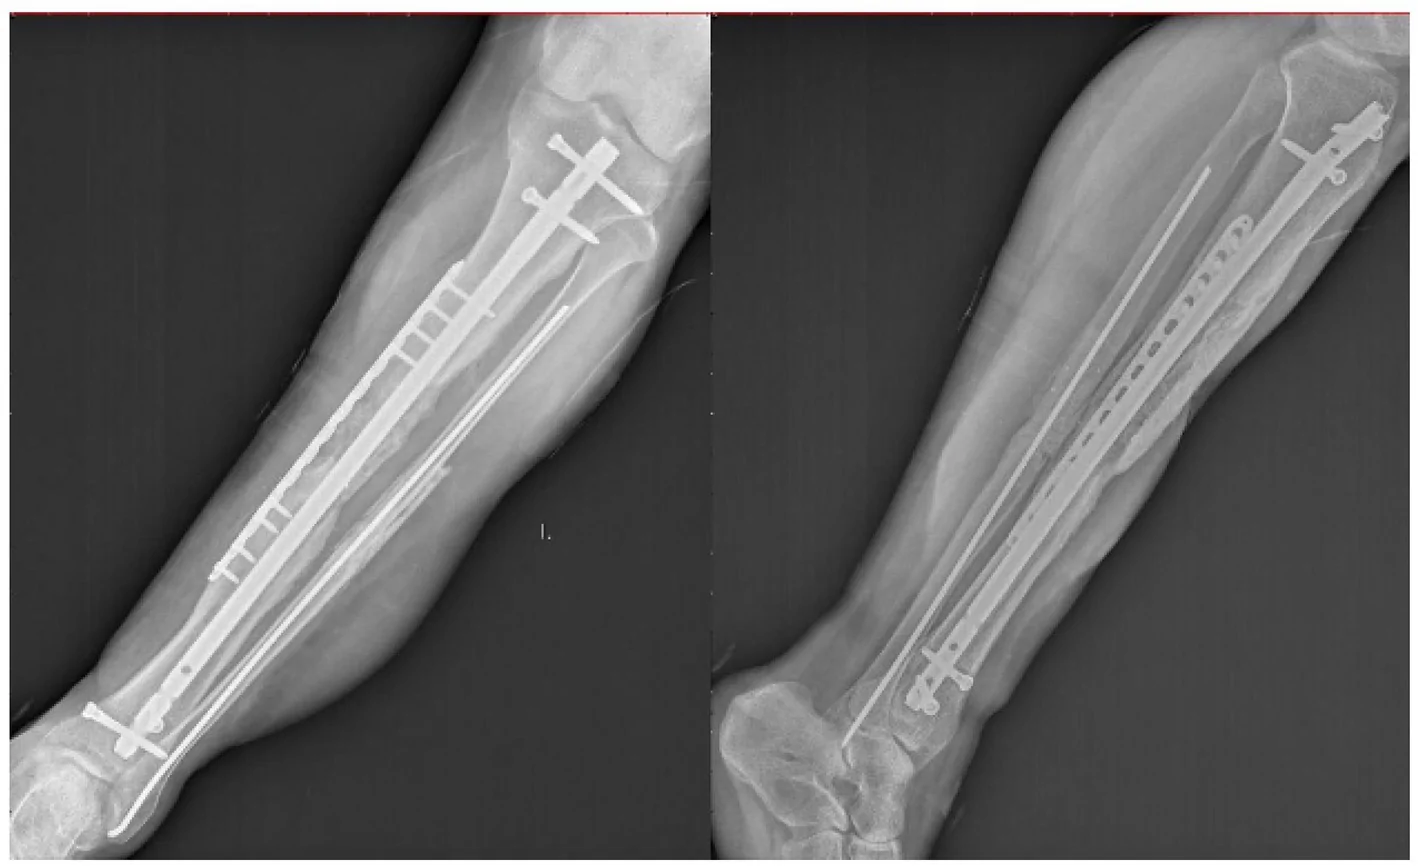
The X-ray findings 14 months after the bone grafting procedure, revealing satisfactory bone healing in the grafted area. The fracture site has largely healed, with evident cortical bone remodeling.
